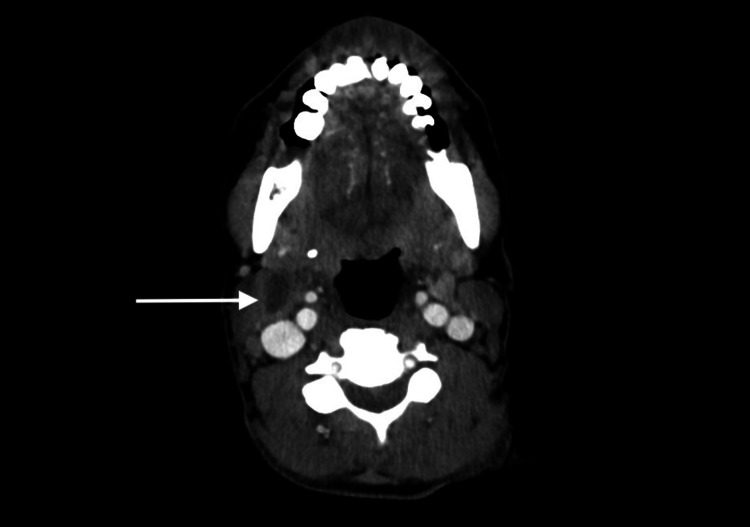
CT scan demonstrating necrotic level IIA lymph node
